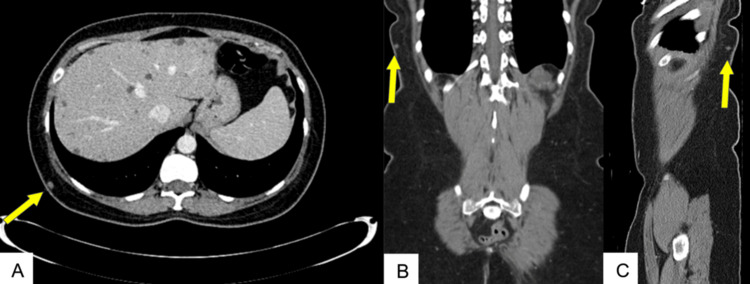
Axial (A), coronal (B), and sagittal (C) CT images from June 2020 show a small, well-defined soft tissue lesion in the right posterolateral chest wall (yellow arrows), initially presumed to represent a benign process such as a lipoma or inclusion cyst.
